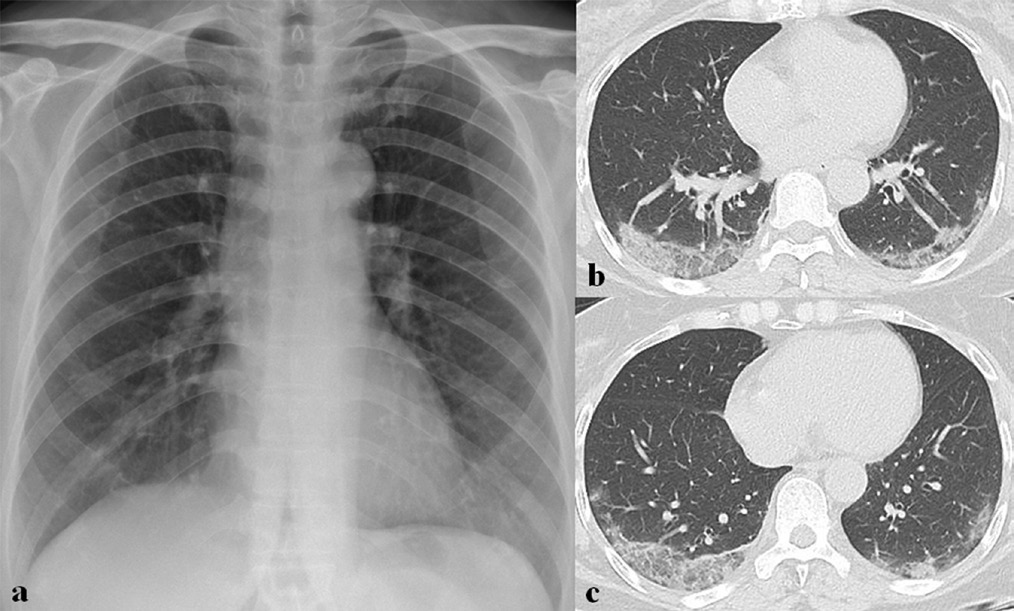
A 64-year-old female patient with SARS-CoV-2 infection. (a) Initial chest radiograph shows faint ground-glass opacities (GGOs) in both lower lung zones. (b, c) Follow-up CT scans obtained 14 days after symptom onset show mixed GGOs and consolidation in both lower lung lobes.
